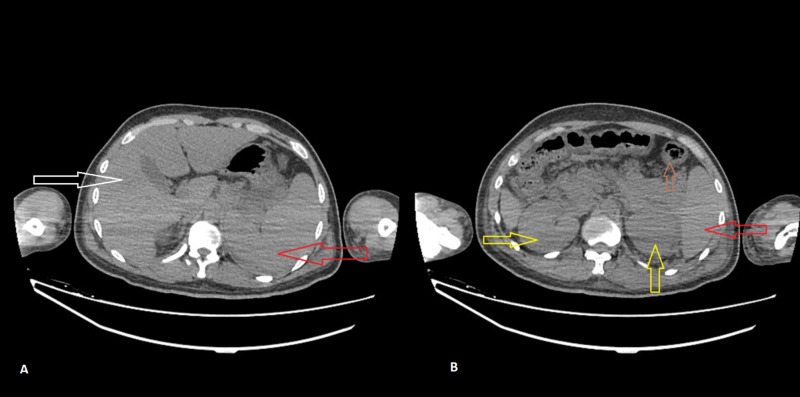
Computed tomography (CT) abdomen without contrast. Image A: White arrow points towards an enlarged liver; red arrow points towards splenomegaly. Image B: Red arrow points towards the inferior portion of the enlarged spleen; yellow arrow points towards swollen and globular appearing kidneys; brown arrow points towards diffuse thickening of the wall of the colon.
